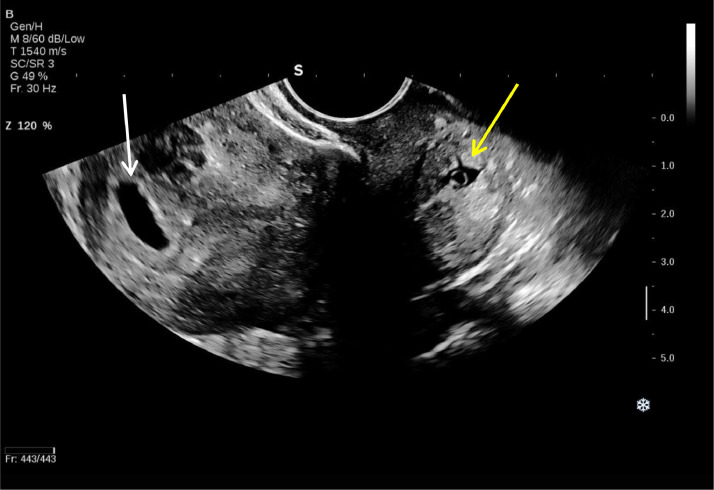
Transvaginal ultrasound revealed a GS in the proximal uterine cavity (white arrow) and a GS in the posterior wall of distal cervix (yellow arrow) suggestive of cervical heterotopic pregnancy.
